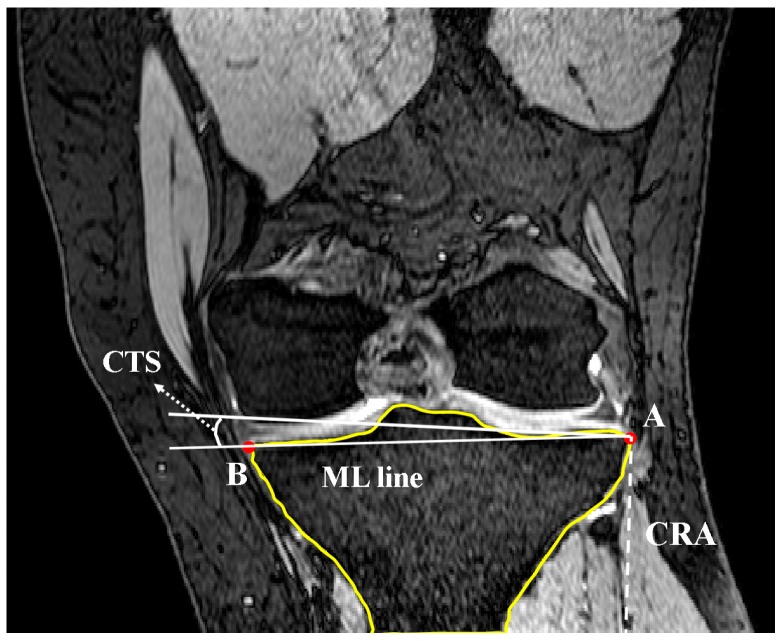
Calculation of coronal tibial slope in interested MRI slice as the angle between ML line and the perpendicular line of CRA (coronal view).
